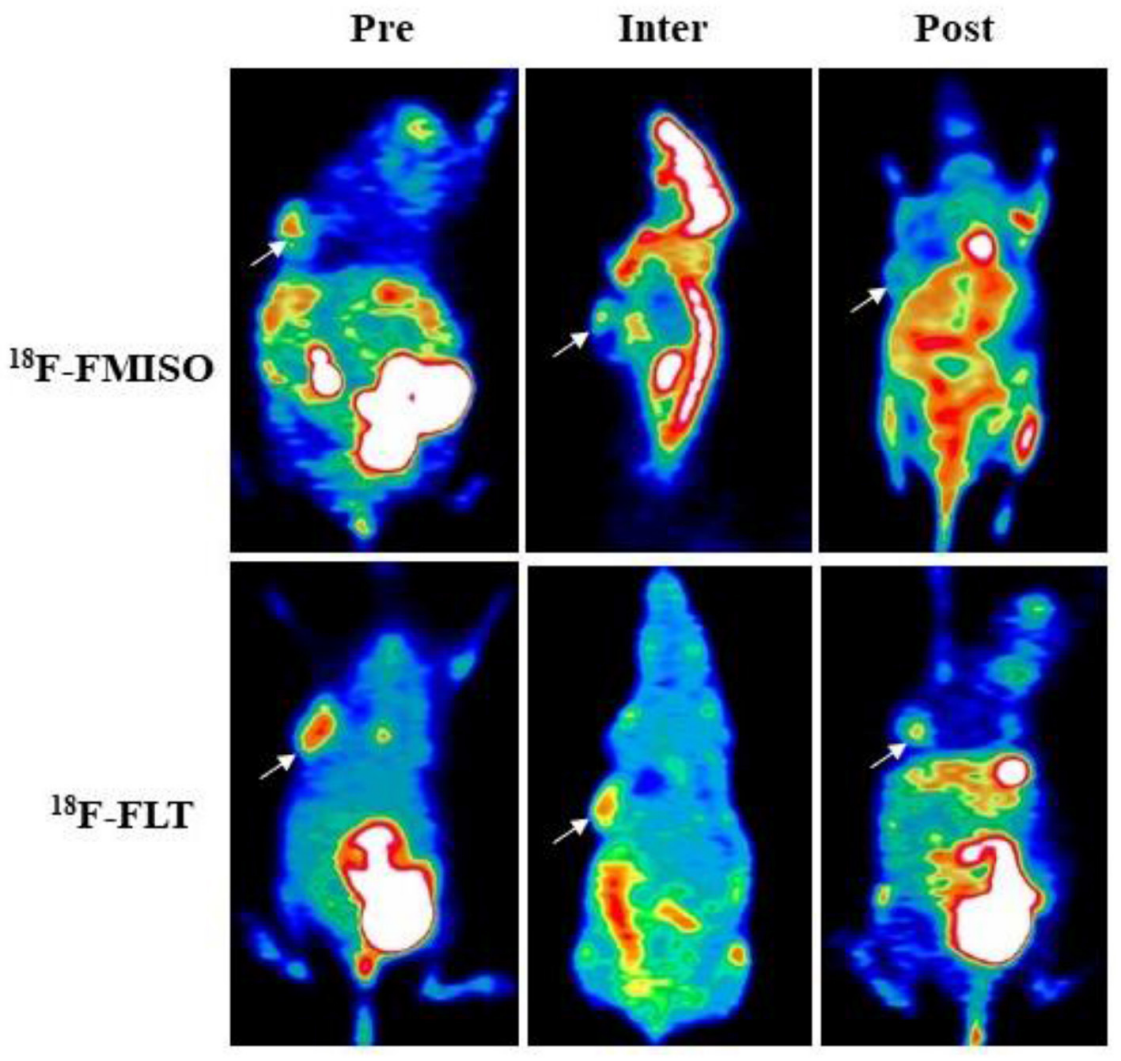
^18^F-FMISO and ^18^F-FLT-based micro-positron emission tomography (PET) detecting FaDu xenografts. White arrows indicate tumor location (Pre, Inter and Post represent data before (0 Gy), during (21 Gy), and after (40 Gy) radiotherapy, respectively). In ^18^F-FMISO PET images, the TNR, SUVmax, and HV were significantly decreased from Pre-FRT to Inter-FRT (*p* < 0.001, *p* < 0.001, *p* = 0.031, respectively) and from Inter-FRT to Post-FRT (*p* = 0.004, *p* = 0.008, *p* = 0.025, respectively). In ^18^F-FLT PET images, SUVmax and PTV were significantly decreased from Pre-FRT to Inter-FRT (*p* = 0.032, *p* = 0.041, respectively) and from Inter-FRT to Post-FRT (*p* = 0.017, *p* < 0.001, respectively).
